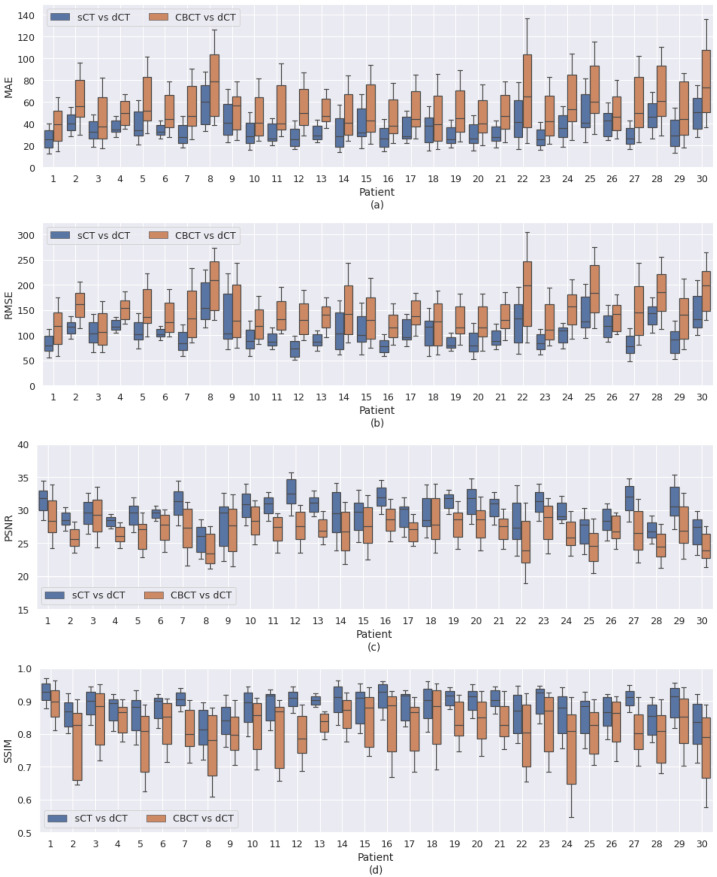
Comparison of sCT with dCT and CBCT with dCT on different similarity metrics, (a) MAE, (b) RMSE, (c) PSNR, and (d) SSIM, for each patient in the test set. Values are calculated across all slices from the same patient.
